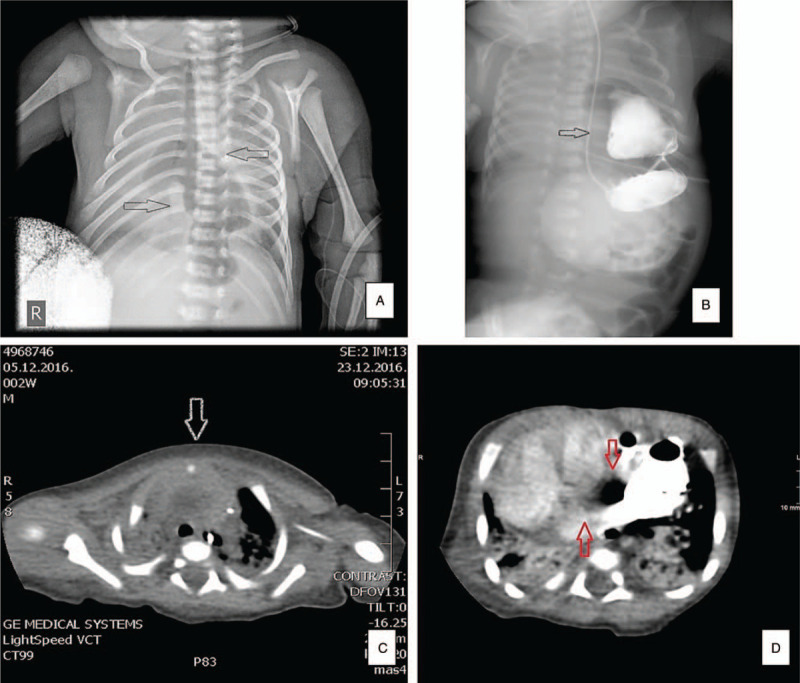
A–D: A: Plain chest X-ray with transparent, air-filled shadow positioned in the midline of the thorax-abnormal position of the stomach (white arrows); B: AP view—a plain X-ray of the upper intestinal tract with hydrosoluble contrast in abnormally intrathoracic positioned stomach (white arrow); C: Axial CT scan indicates one small ossification center of the sternum (incomplete ossification of the sternum) (white arrow); D: Coronal CT scan where is shown a close connection between pericardial sac and stomach wall (stomach filled with an oral contrast) (red arrows). CT = computed tomography.
